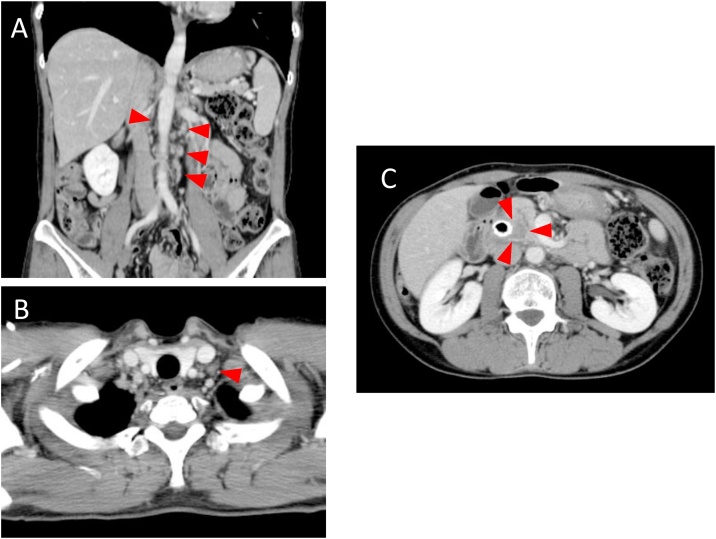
Computed tomography (CT) scan after FOLFIRINOX (combination of 5-fluorouracil, leucovorin, irinotecan, and oxaliplatin) treatment showing only tiny appearances of distant lymph nodes. A: para-aortic lymph nodes (red arrow heads); B: left supraclavicular lymph node (a red arrow head); C: primary lesion (red arrow heads).
